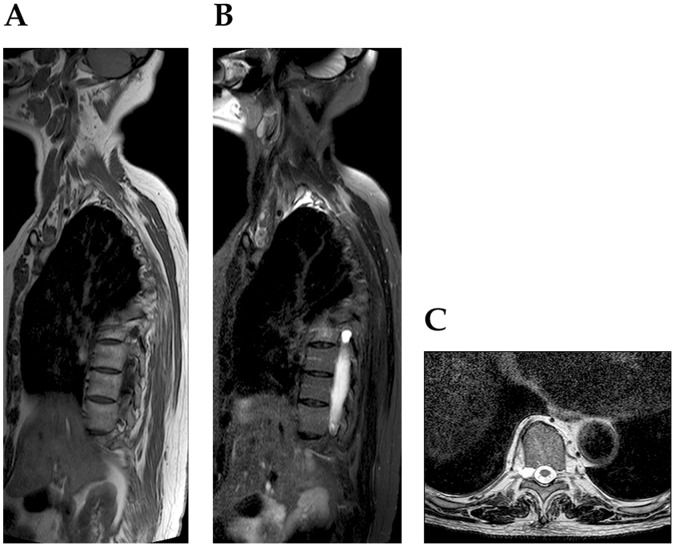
60-year-old woman with a perineurial cyst in the right T6 intervertebral foramen (between T6-T7), other cysts are not visible due to scoliosis. Sagittal section on T1- (A) and T2-weighted (B) images. Axial T2-weighted (C) image.
